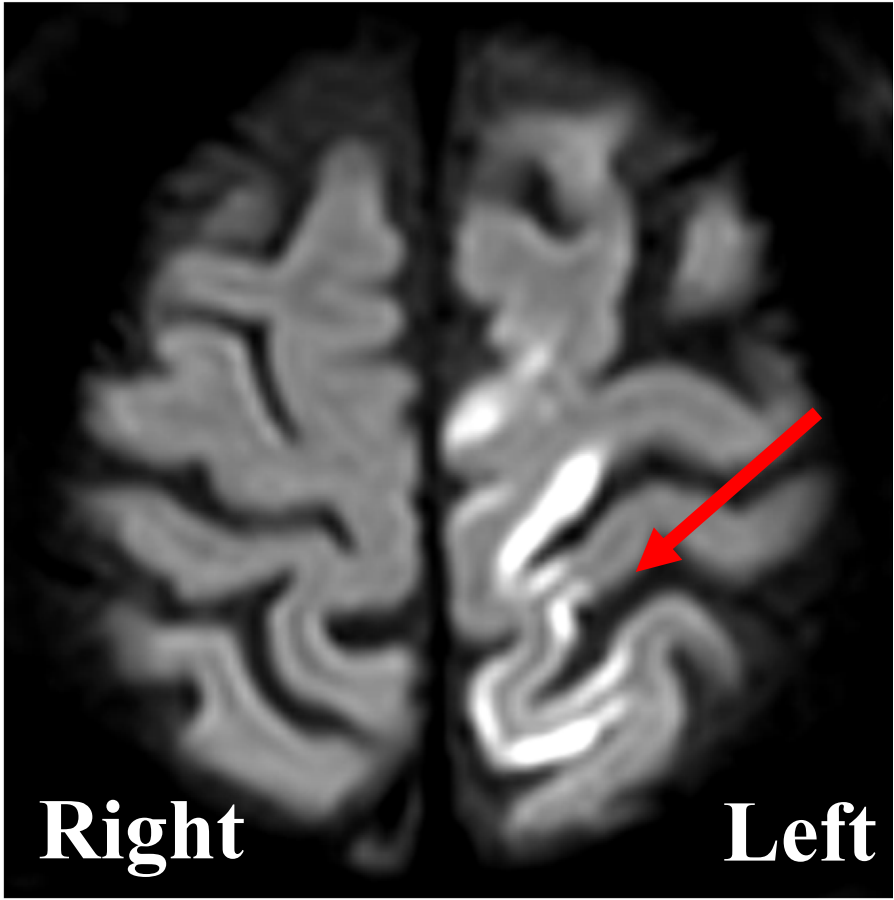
Postoperative T2-weighted magnetic resonance imaging of the brain. The red arrow indicates a high-density presence at the left frontal lobe
